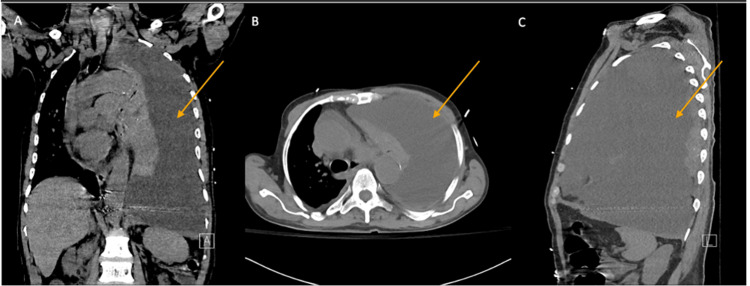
Chest CT demonstrating large left pleural effusion resulting in complete ipsilateral lung collapse.
